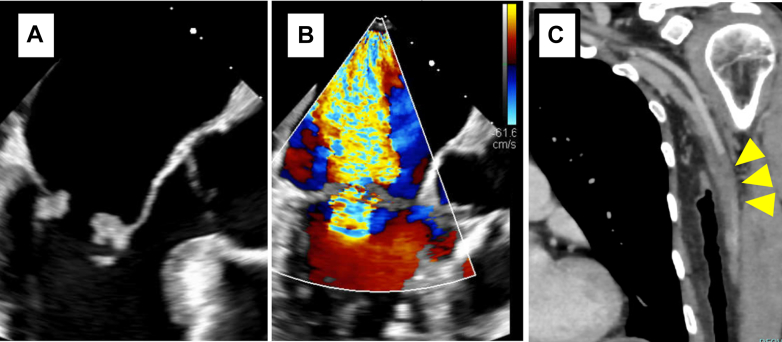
TEE and Contrast-Enhanced CT Findings After Recurrent NBTE (A and B) TEE revealed vegetations on both mitral valve leaflets, with the largest measuring 9 × 7 mm on the anterior leaflet. These findings were accompanied by severe mitral regurgitation. (C) Contrast-enhanced CT demonstrated occlusion of the left brachiocephalic artery (arrowheads). CT = computed tomography; NBTE = nonbacterial thrombotic endocarditis; TEE = transesophageal echocardiography.
